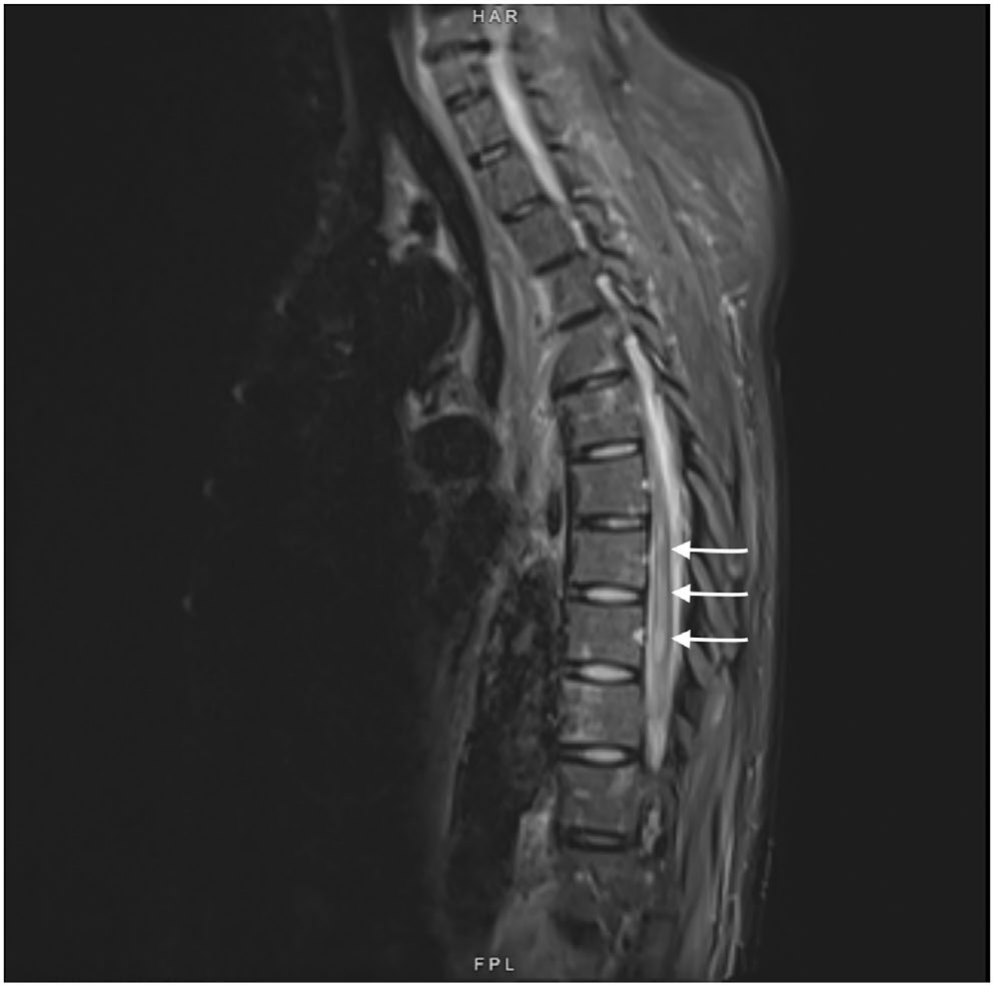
MRI T2 sagittal view of thoracic spinal cord. There is abnormal T2 hyperintensities (white arrows) in T7‐T9 spinal cord suggestive of cord infarction.
